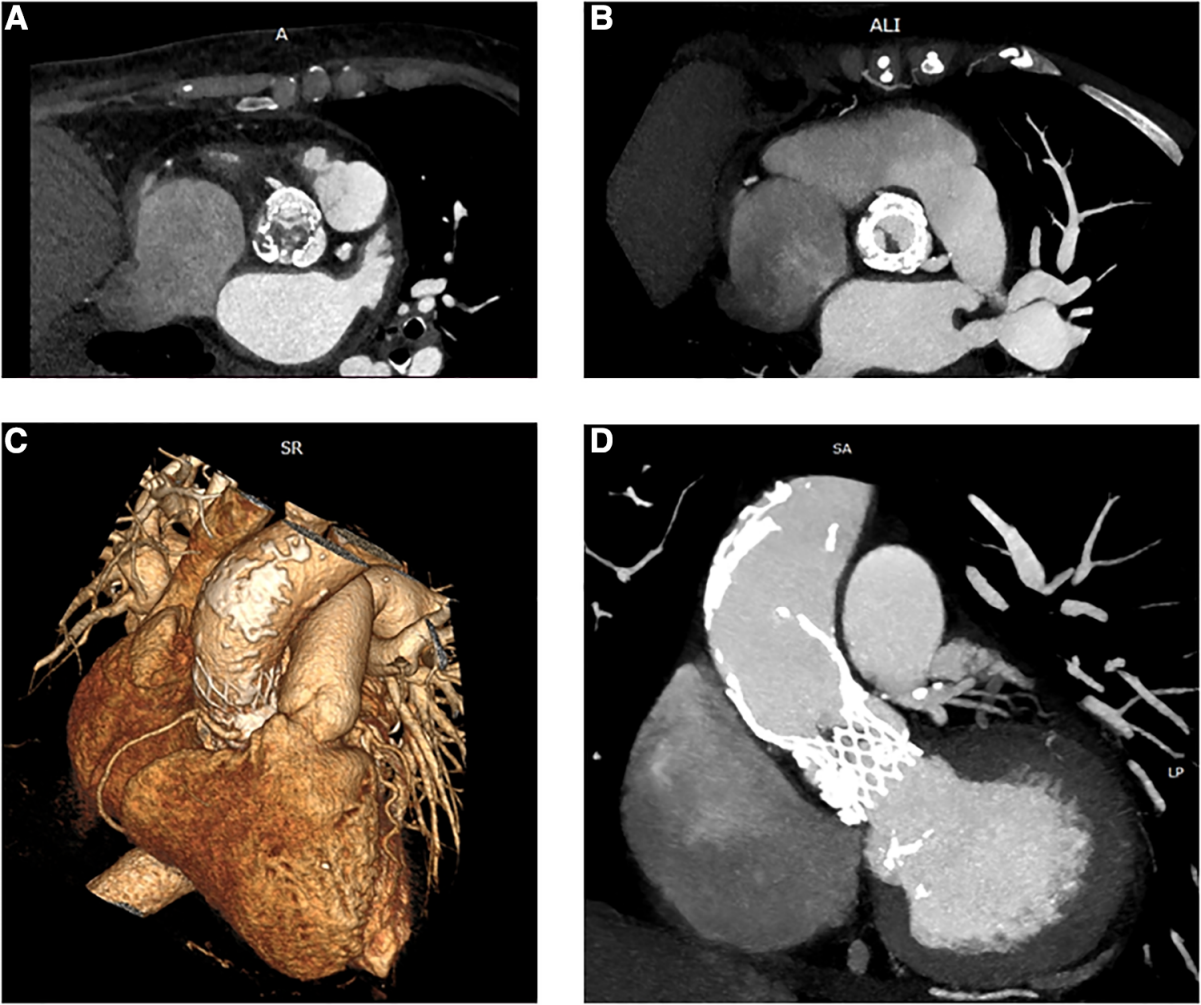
Preoperative CT: axial view of the CoreValve prosthesis with vegetations in the left (A) and right (B) coronary cusps. 3D reconstruction of the ascending aorta with features of porcelain aorta (C). Multiplanar reconstruction (MPR) shows thrombosis and vegetation inside the CoreValve scaffold and prominent calcification of the aorta (D).
